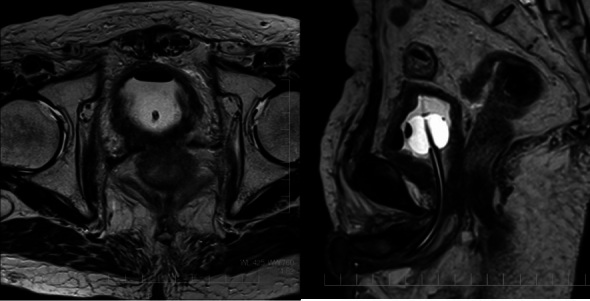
Pelvic MRI with urinary catheter shows significant reduction of the multiple
